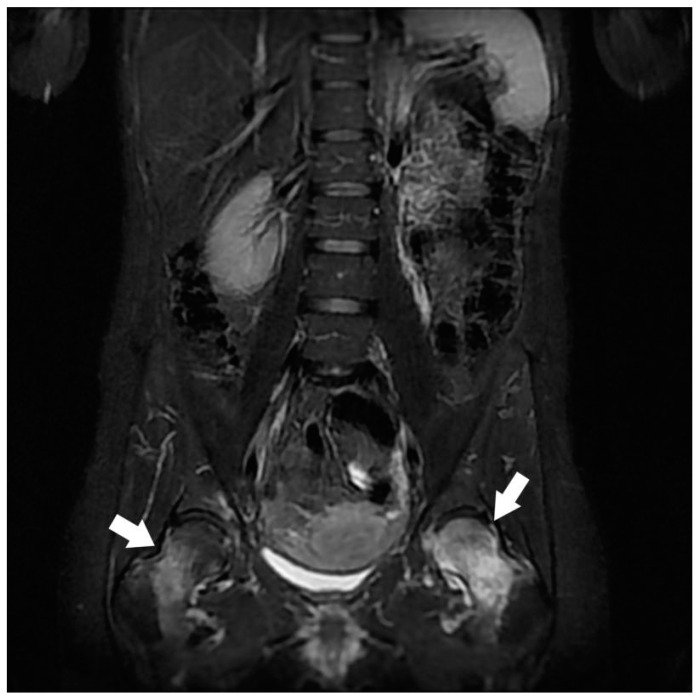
Assessment of bilateral sciatica in a 40-year-old woman in a postpartum context. Coronal STIR MRI. Bilateral hypersignal essentially involving the proximal ends of the femurs (white arrows) and, to a lesser extent, the bottom of the acetabulum. Predominant involvement on the left with joint effusion. Respect of the coxo-femoral joint spaces. Transient migrant regional osteoporosis.
